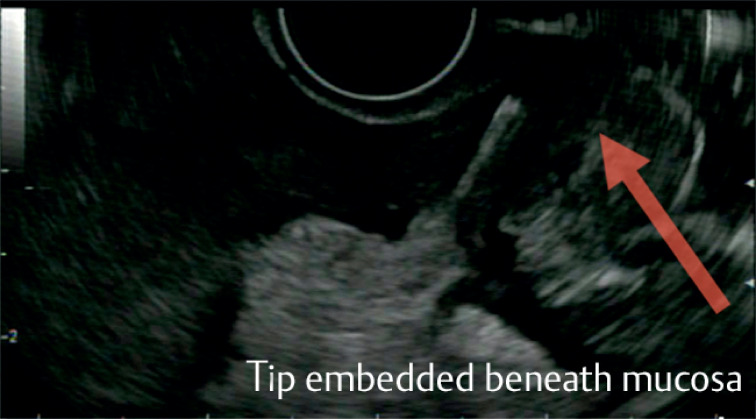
On endoscopic ultrasound, the tip of the foreign body was seen embedded beneath the mucosa.
